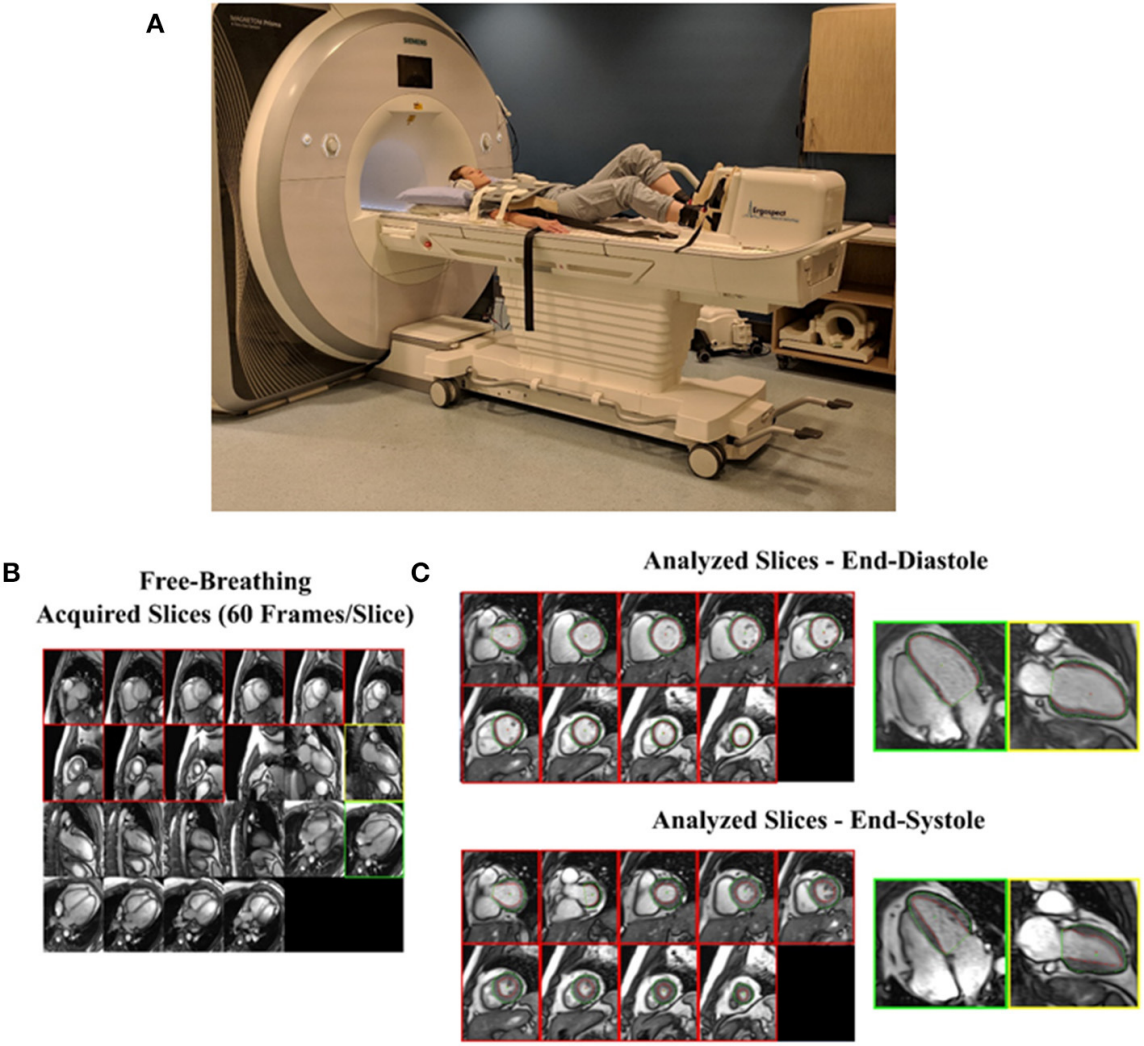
Real-time imaging of cardiac function during exercise using cardiac MRI. (A) Healthy volunteer in supine position outside of magnet bore using MRI conditional stepper device to achieve maximal aerobic activity. (B) All short-axis and long-axis slices are viewed simultaneously to select those for volumetric analysis. Short-axis slices with myocardium and a single 2- and 4-chamber view are chosen. (C) A full cardiac cycle for each selected slice is extracted from which end-diastolic and end-systolic images are identified and endocardial (red) and epicardial (green) borders are traced. Modified from Kirkham et al. (97).
